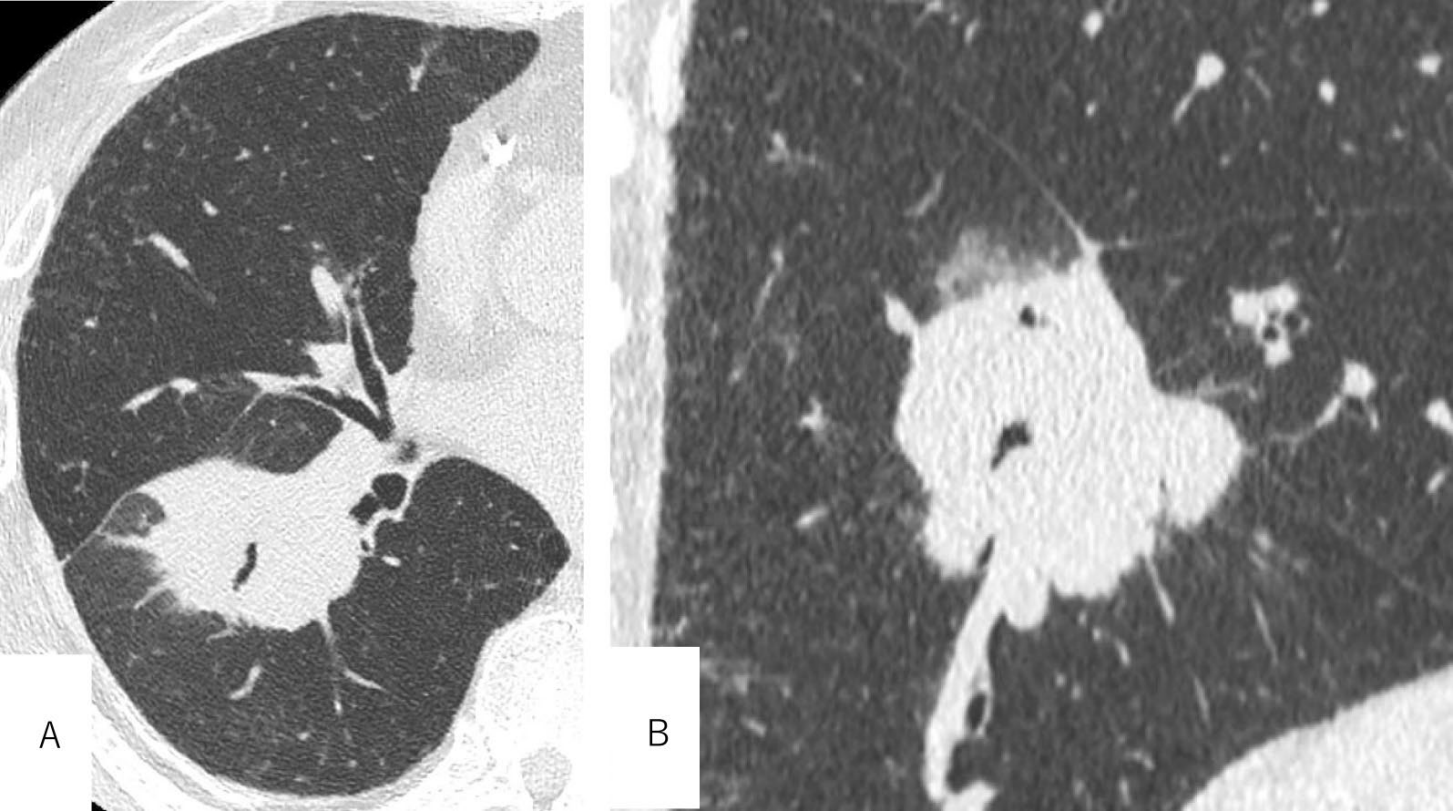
A The initial CT showed a lung mass infiltrating the interlobar PA in the right lower lobe. B A sagittal view revealed that the lung mass was located in the basal segment
